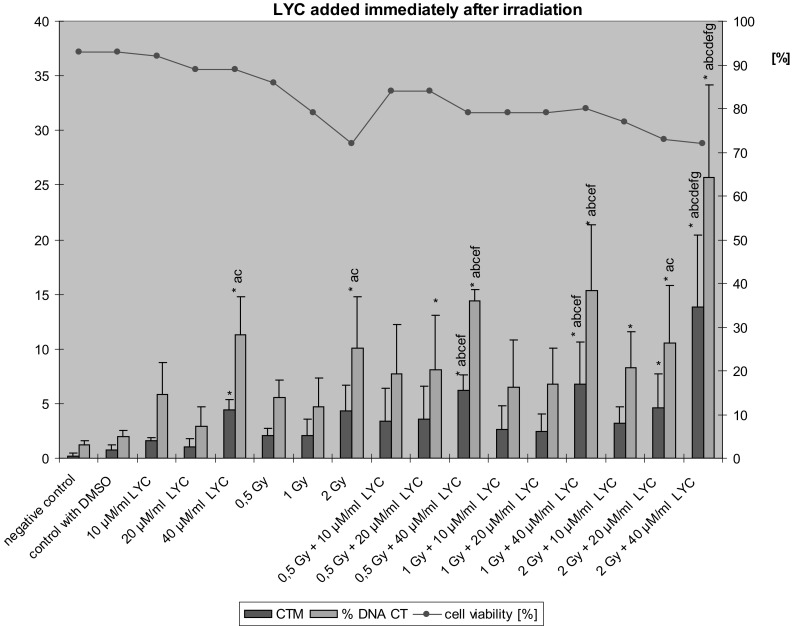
The value of Tail Moment (CTM) and Tail DNA  % (% DNA CT) in lymphocytes treatment by LYC immediately after the exposure to ionizing radiation (*n* = 3); p* < 0.05 compared to control; ^a^
